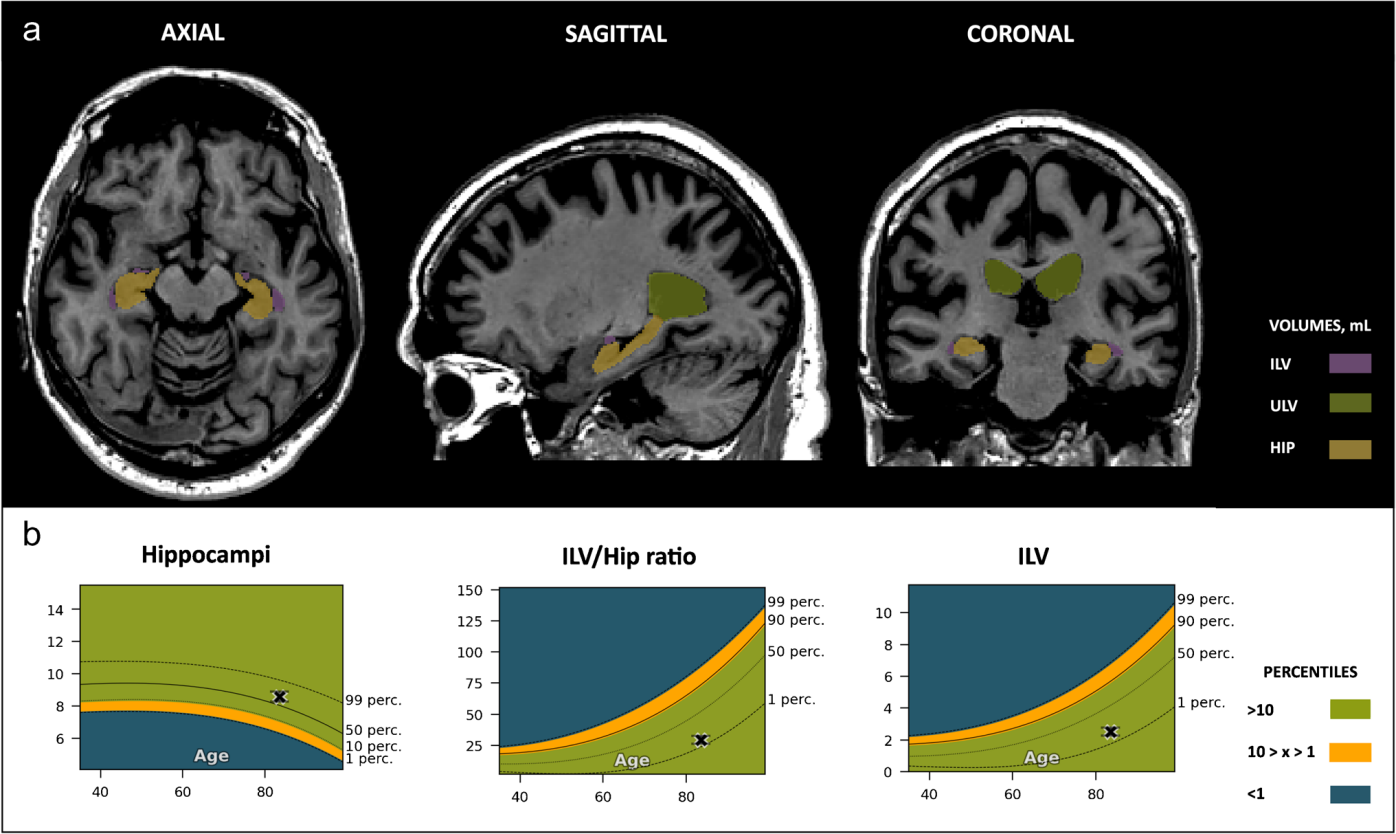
Cognitively normal (CN) case. Cross-sectional coronal T1-weighted image at the level of the medial temporal lobe and hippocampus with segmentation of the upper lateral ventricles (green), inferior lateral ventricle (purple) and hippocampus (yellow). A — Automated volumetric measurements *left*: Axial view *middle*: Sagittal view *right*: Coronal view. B — Population graphs *left*: hippocampal volumes of this subject showing the gray “x” marker in the green region just above between the 50th and 90th percentiles; *middle*: the ILV/Hip ratio of this subject showing the gray “x” marker in the green region between the 1st and 50th percentiles. *Right*: ILV volumes of this subject showing the gray “x” marker in the green region between the 1st and 50th percentiles. Color-coded population graph percentile interpretation; 1–90th percentiles, green (normal); 90–99th percentiles, orange (caution); and > 99th percentile, dark blue (abnormality)
